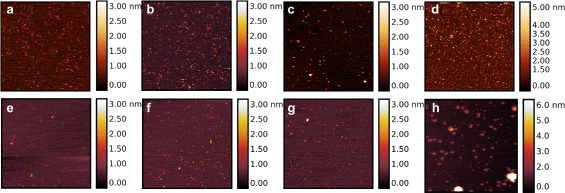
AFM imaging of *β*-Amylase using tapping mode in liquid environment. *Top row* (a, b, c, d) corresponds to images obtained when *β*-Amylase was deposited on Nickel pre-treated mica whereas the *bottom row* (e, f, g, h) corresponds to bare mica. Non-irradiated *β*-Amylase is shown in (a, e) whereas increasing exposure to X-ray beam is shown in (b, f) for 10 s exposure, (c, g) 5 min exposure, (d, h) 30 min exposure. The scan size is 1 *μ*m with each line made of 512 pixels. A clear increase in height (and diameter) can be easily seen upon increase exposure (radiation damage) with no apparent differences between control and standard exposure (10 s) time. A total of 13 AFM images have been used for statistical analysis representing a total of 3693 and 948 particles measured on nickel pre-treated mica and bare mica, respectively
